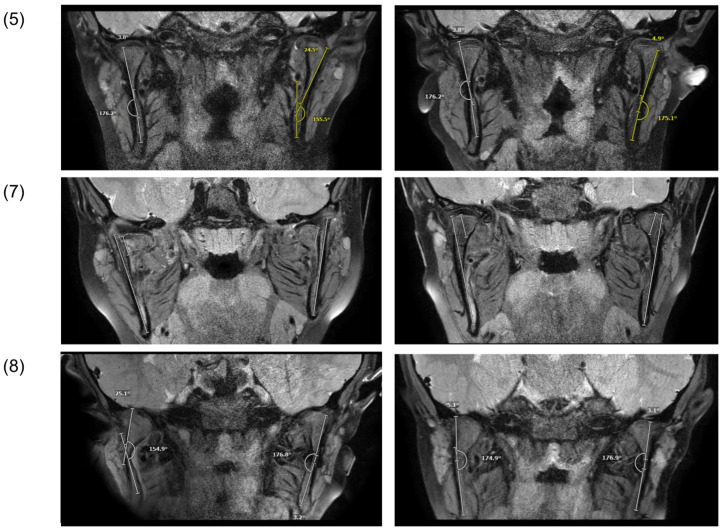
Magnetic resonance image of patients after trauma (t_0_, left) and after treatment (t_1_, right).
